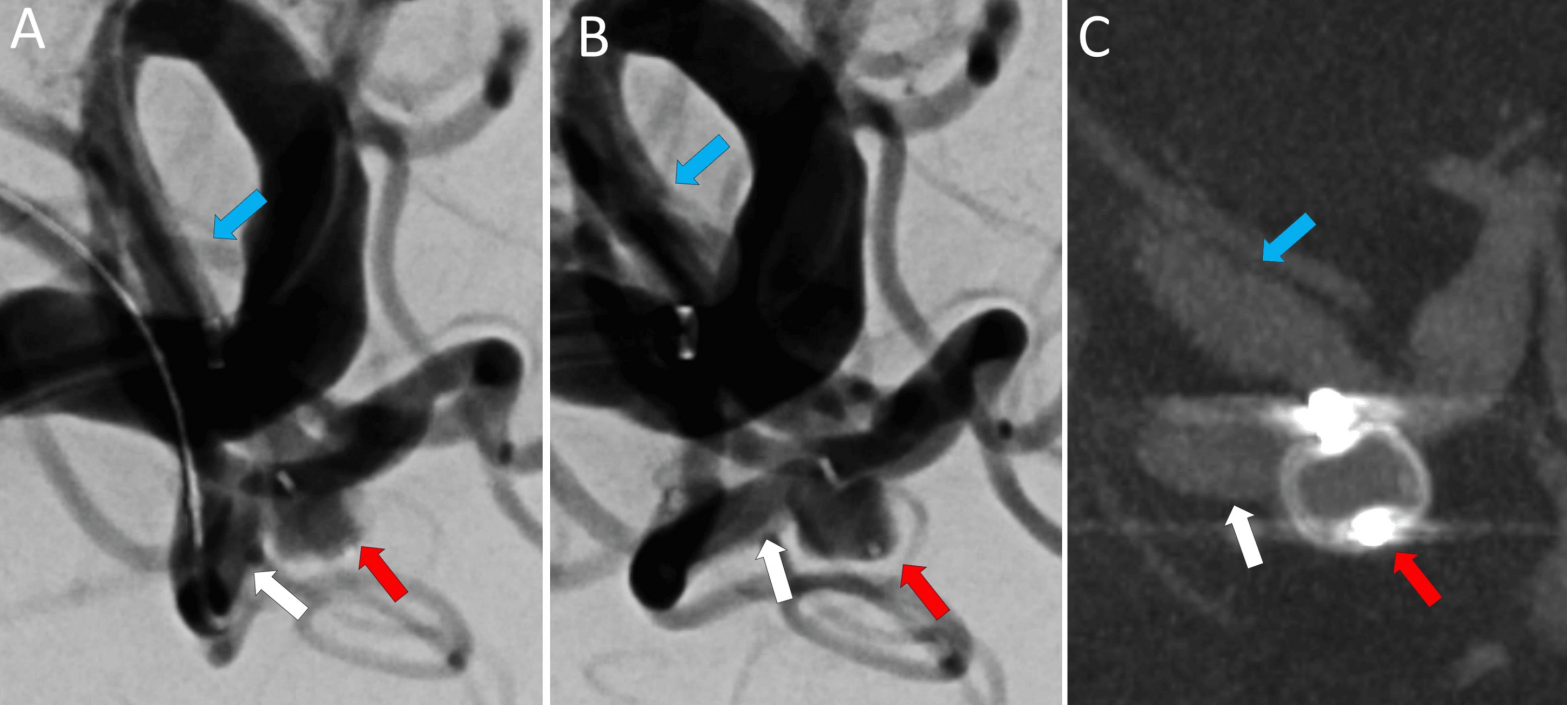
Angiograms of WEB deployment. A: The Woven EndoBridge (WEB) was deployed within the middle cerebral artery (MCA) aneurysm. B: After removing the stiff microguidewire, the modified angle returned to its original position. C: High-resolution cone-beam CT also confirmed the appropriate position of the WEB. The red arrow indicates the MCA aneurysm, the white arrow points to the M2 inferior trunk, and the light blue shows the M1 trunk.
